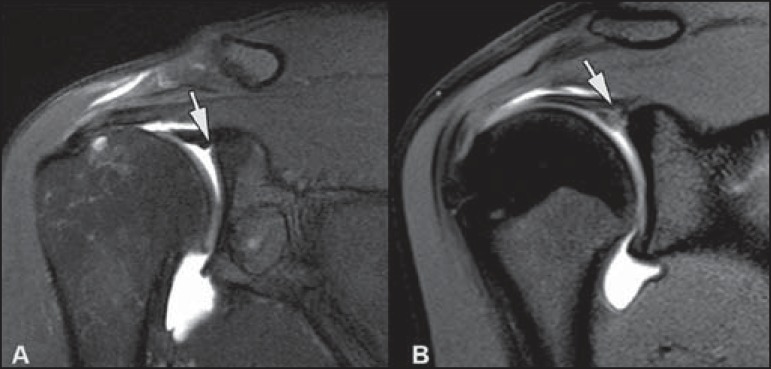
Coronal MR arthrography T1-weighted sequence showing a normal sulcus in the region
